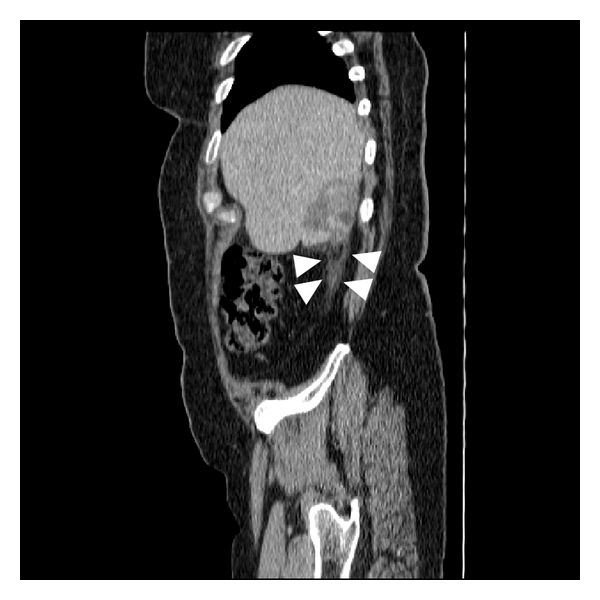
Reimaging of the abdomen with computed tomography three days subsequent to the original study in the coronal plane exhibits an increase in the amount of paracolic gutter fluid (arrowheads), and the craniocaudal dimension of the hemangioma is now 73.8 mm.
